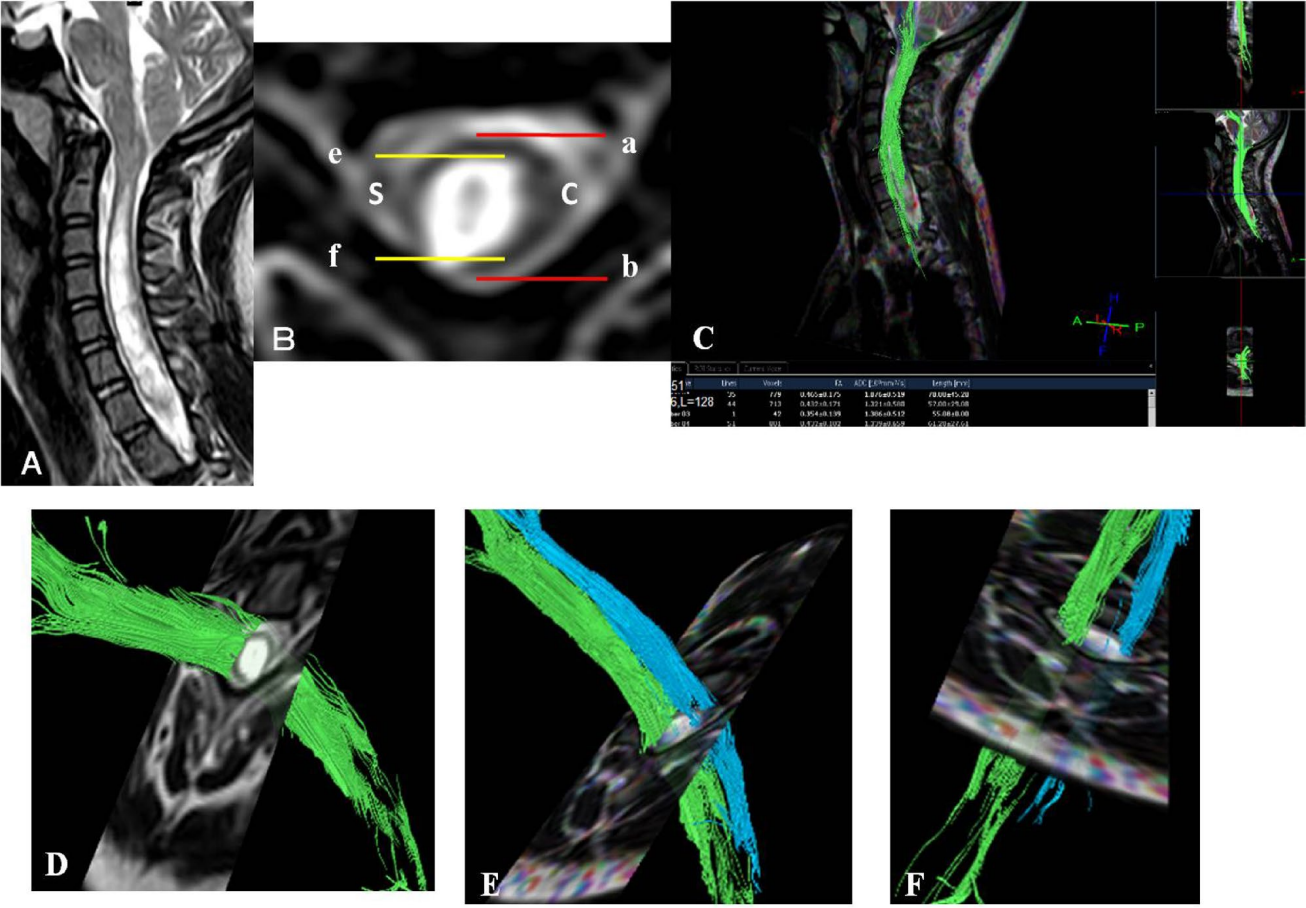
(A) MRI image at sagittal plane on T2W, (B) the ratio as index of syrinx dimension which was calculated by maximal syrinx diameter (S)/spinal cord diameter (C) at the same location on T2W axial. (C) Location map and measurement of DTI parameters on sagittal plane. (D–F) Measurement of DTI parameters on transverse plane.
